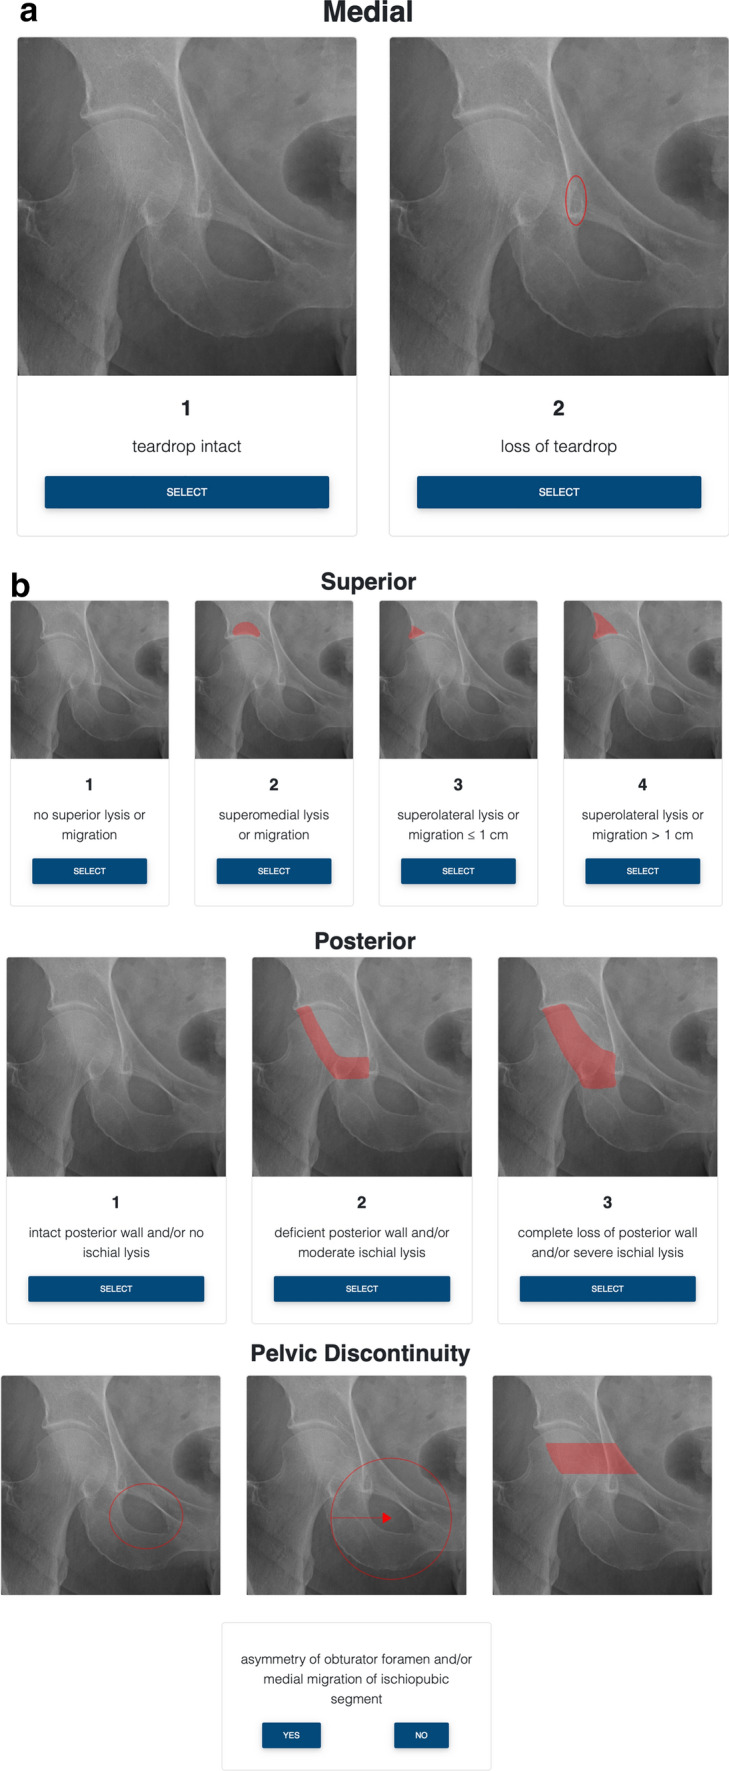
The ADC application is designed as a walk-through, guiding the end user through a radiographic evaluation of an acetabular defect. Due to the condensation of information by depicting a complex, three-dimensional bone defect on a two-dimensional native radiograph, structured and detailed evaluation is essential to achieve a reliable grading. In all grading steps a native a.p. radiograph of a right-sided, healthy hip joint is provided for comparison. a Beginning with the medial portion of the acetabulum, the end user can evaluate the integrity of the “teardrop”, a radiographic phenomenon resulting in the overlay of different bone surface encompassing the medial wall. The teardrop is marked with a red oval ellipse. For options, either “teardrop intact” or “loss of teardrop” can be selected. b In the next grading step, the superior aspect of the acetabular rim can be evaluated. The region of interest is clearly marked by a red color overlay. Options extend from “no superior lysis or migration”, over “superomedial lysis or migration”, and “superomedial lysis or migration < 1 cm”, to “superolateral lysis or migration > 1 cm. c On the following page the posterior aspect of the acetabulum is evaluated. The region of interest is clearly marked by a red color overlay. Possible choices are: “intact posterior wall and/or no ischial lysis”, “deficient posterior wall and/or moderate ischial lysis” and “complete loss of posterior wall and/or severe ischial lysis”. d At the last grading step direct or indirect signs of pelvic discontinuity need to be assessed. If any signs of pelvic discontinuity are present, such as “asymmetry of obturator foramen and/or medial migration of ischiopubic segment” (compare to the first and second marked image) or a visible fracture line can be observed (compare to the third marked image), the user can choose “yes” and continue
